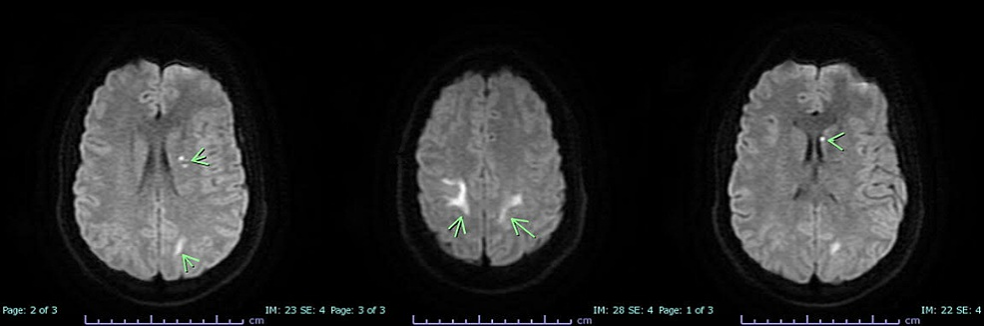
MRI of the brain showing cerebrovascular accident of posterior parietal lobes bilaterally and left basal ganglia
